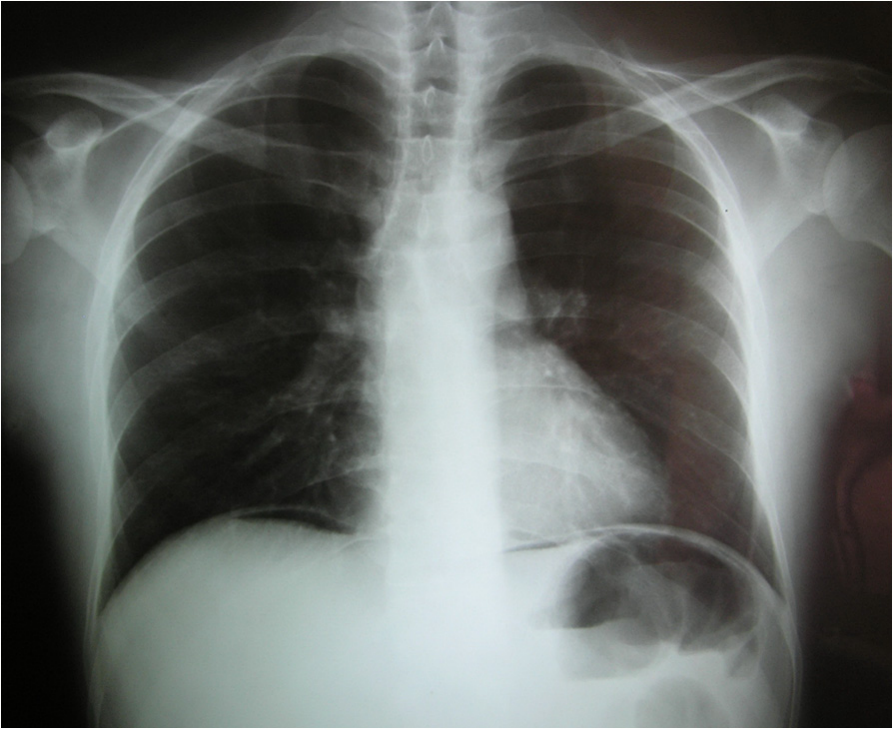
Chest X-ray revealing pneumoperitoneum.
